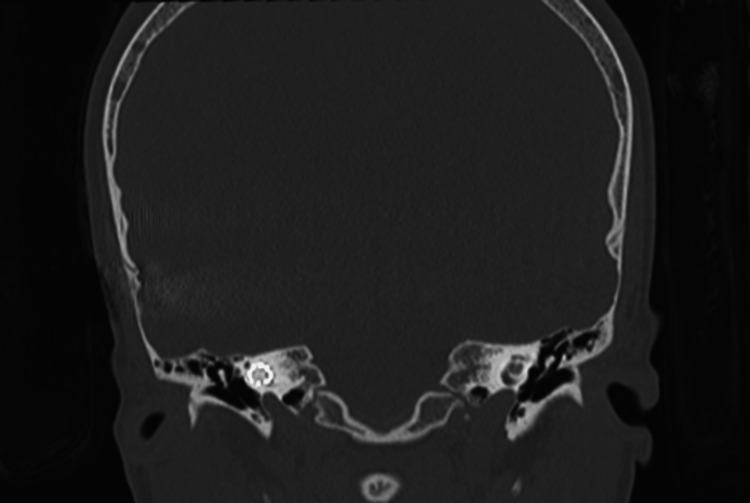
CT temporal bone coronal view of the same patient showing right cochlear labyrinthine ossificans.
